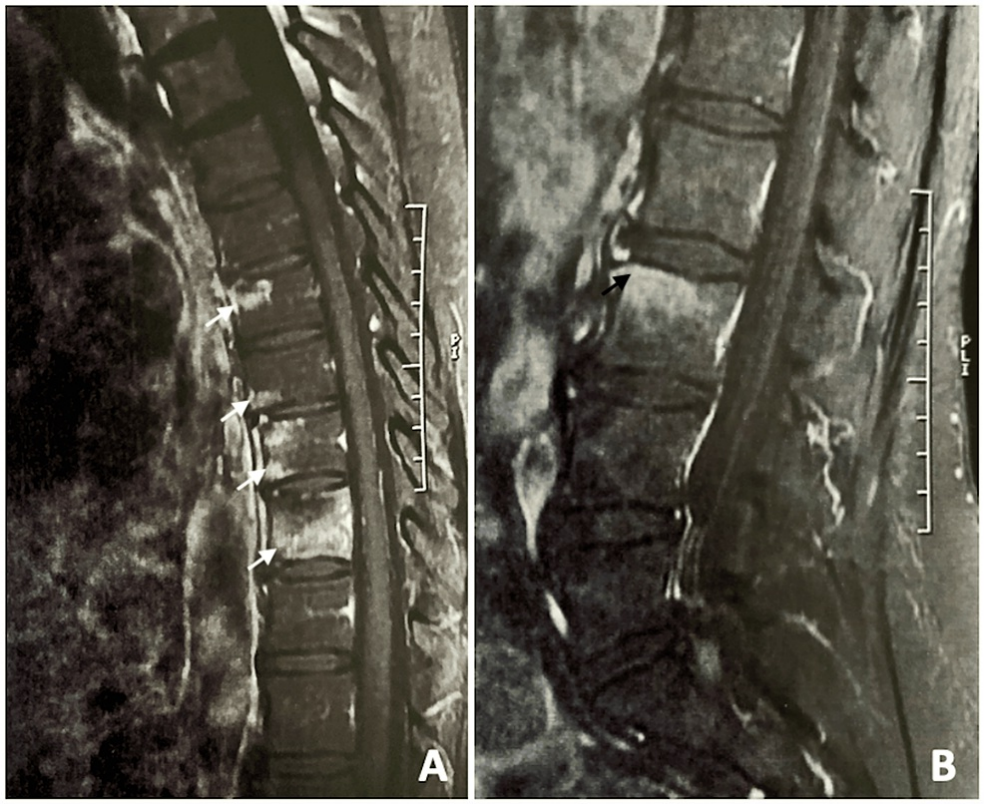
Gadolinium-enhanced sagittal T1-weighted MRI revealing contrast enhancement at the vertebral bodies of T7, T8, T9, T10 (white arrows) (A), and L3 (black arrow) (B).
